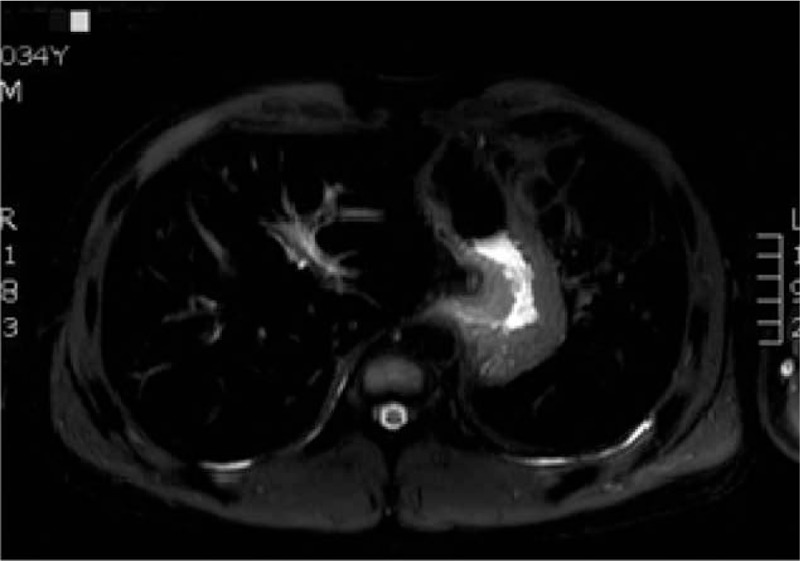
The signal of liver reduced on T2W1, and represented as a “black liver” on MRI scan. MRI = magnetic resonance imaging.
